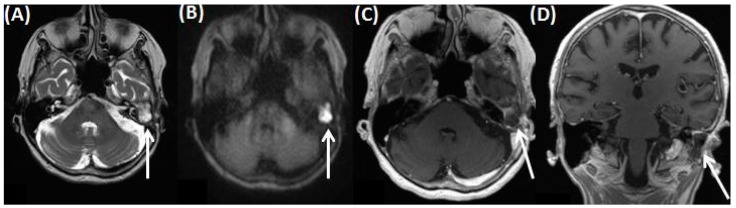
MRI of an 80-year-old female patient with diagnosis of a cholesteatoma of the left EAC after mastoidectomy at this side. Cholesteatoma can be detected as hyperintense on T2-weighted images (A), it appears hyperintense on DWI (B), and can only be clearly delineated on T1-weighted images after contrast agent application as shown in (C,D).
